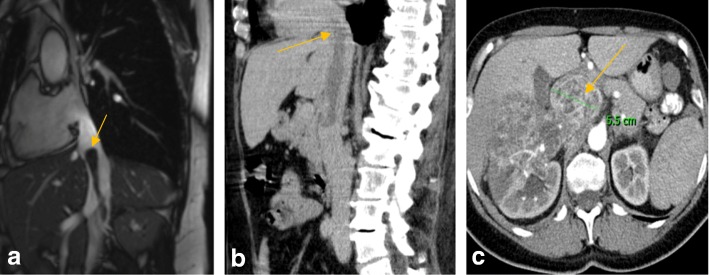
Preoperative computed tomography/magnetic resonance imaging of the renal carcinoma malignant tumorous thrombus level IV infiltration of the inferior vena cava (*arrow* marking the tumorous thrombus). a Patient 1: supradiaphragmatic tumorous thrombus without the right atrium infiltration. b Patient 2: suprahepatic tumor thrombus. c Patient 3: right kidney tumor with dilated inferior vena cava up to 5.5 cm
